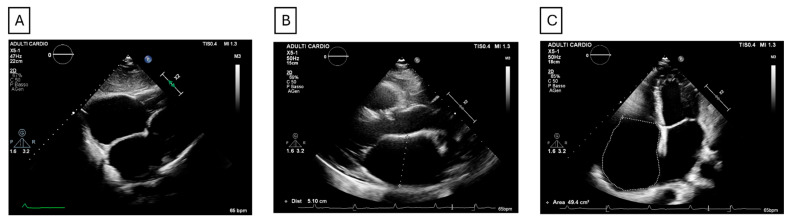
Echocardiographic images representative of atrial cardiomyopathy. (A) Subcostal view showing massive atrial enlargement and absence of pericardial effusion. (B) Parasternal long-axis view demonstrating left atrial dilation (antero-posterior diameter 51 mm). (C) Apical four-chamber view showing a right atrial area of approximately 50 cm^2^.
